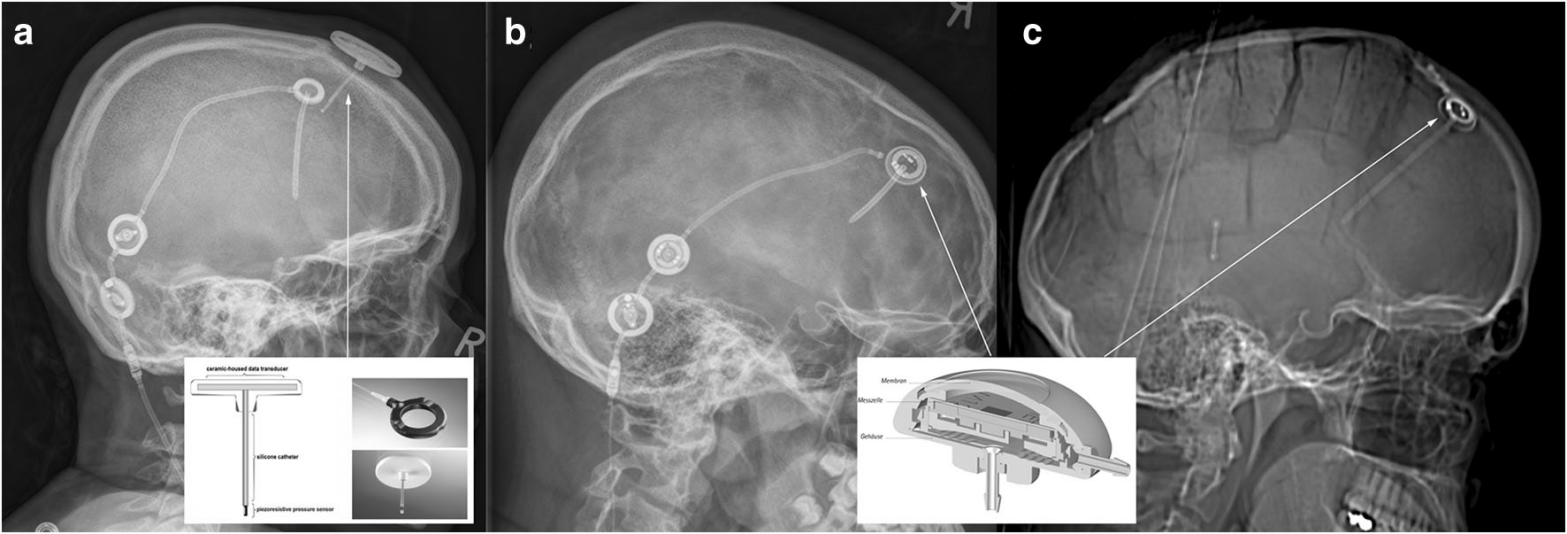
Representative x-rays of the different set ups: a AP view on a Neurovent P-tel device on the left (*) and shunt system on the right with a proV-proSA-GA valve complex. b Lateral view on a Sensor Reservoir connected with proV-proSA-GA valve complex. In both the proSA was placed in between the two components of a proGAV valve. c Lateral view on a Sensor Reservoir as stand-alone device. *proV* programmable DP valve, *proSA* programmable shunt assistant, *GA* gravitational assistant unit, *proGAV* programmable DP valve with gravitational assistant unit
